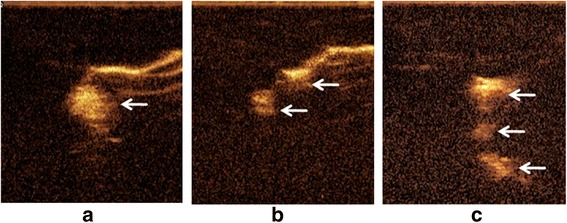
Enhanced lymph nodes (*single arrows*) on contrast-enhanced ultrasound. a Only one lymph node enhanced. b Two sequentially visualized SLNs linked by lymph vessel. c Three ordinally enhancing SLNs in a row
